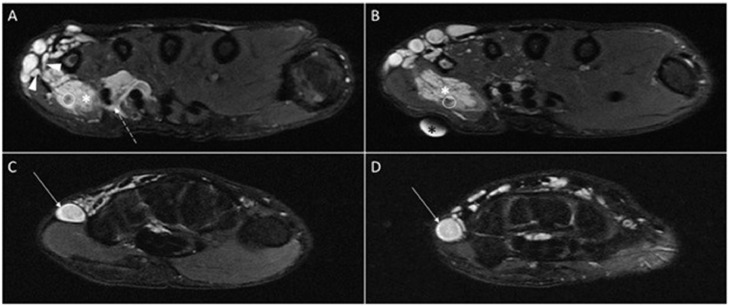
Axial fat-suppressed proton density (PD) MRI of hand and wrist. (A, B) A lobulated hyperintense mass (white asterisk) is seen in the hypothenar region at the site of lump palpation (black asterisk indicates the body marker). Peri and inter tendinous growth are noted around the fourth and fifth flexor digitorum superficialis and profundus tendons (dashed arrow). The mass contains internal areas of hypo signal intensity (circles) indicating phleboliths. Dilated tortuous draining veins are seen medially (arrowheads). (C, D) The aneurysmally dilated basilic vein is seen in the medial aspect of the forearm with a turbulent flow (arrow). Engorgement of other adjacent superficial veins is noted as well.
